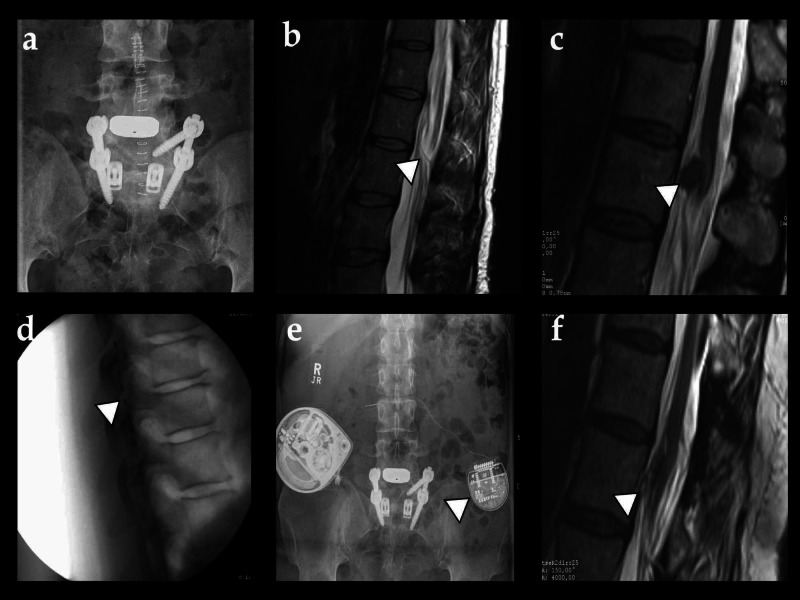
Image series a) Lumbar PA X-ray of the patient after undergoing lumbar fusion L4-L5 and, later on, extended fusion L4-S1. b) T2-weighted MRI scan of the thoracolumbar transmission in the sagittal view showing the catheter for intrathecal morphine therapy within the spinal canal (arrowhead). c) After the patient-reported new-onset left-sided radicular pain, an MRI scan revealed a tumorous formation associated with the catheter tip and the conus terminalis (arrowhead). d) Lateral view image of the intraoperative fluoroscopy of the SCS epidural electrode implanted in the patient. e) Abdominal PA X-ray showing the implanted neurostimulator (device on the left, arrowhead) and the IADP (device on the right). f) A late T2-weighted MRI scan revealed a significant reduction of the granuloma size after the progressive reduction of the morphine dose in the pump and SCS therapy PA: posteroanterior; MRI: magnetic resonance imaging; SCS: spinal cord stimulation; IADP: intrathecal analgesic delivery pump
